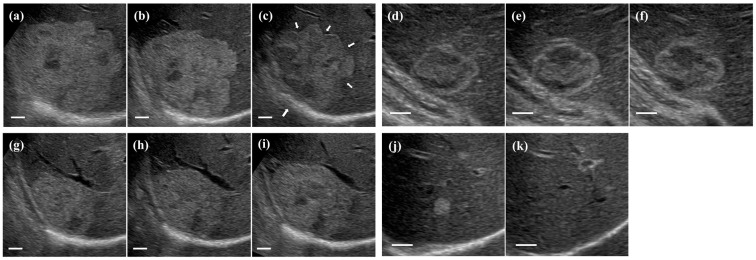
Sonographic changes in size of hepatic hemangiomas. (a–c) Longitudinal sonograms of a hepatic hemangioma in a patient with decreased tumor size. The initial sonogram (a) and follow-up sonograms obtained 39 (b) and 81 months (c) after the initial diagnosis. Retraction of the liver capsule is seen adjacent to the tumor (large arrow), and concavity of the tumor margin becomes more marked with time (small arrow). (d–f) Longitudinal sonograms of a hepatic hemangioma in a patient with no change in tumor size. The initial sonogram (d) and follow-up sonograms obtained 33 (e) and 77 months (f) after the initial diagnosis. (g–i) Longitudinal sonograms of a hepatic hemangioma in a patient with increased tumor size. The initial sonogram (g) and follow-up sonograms obtained 42 (h) and 79 months (i) after the initial diagnosis. (j,k) Longitudinal sonograms of a hepatic hemangioma in a patient in whom the tumor disappeared. The follow-up sonogram obtained 79 months (k) after the initial diagnosis shows no evidence of the hemangioma in the previously noted region (j). White bars indicate a length of 10 mm.
